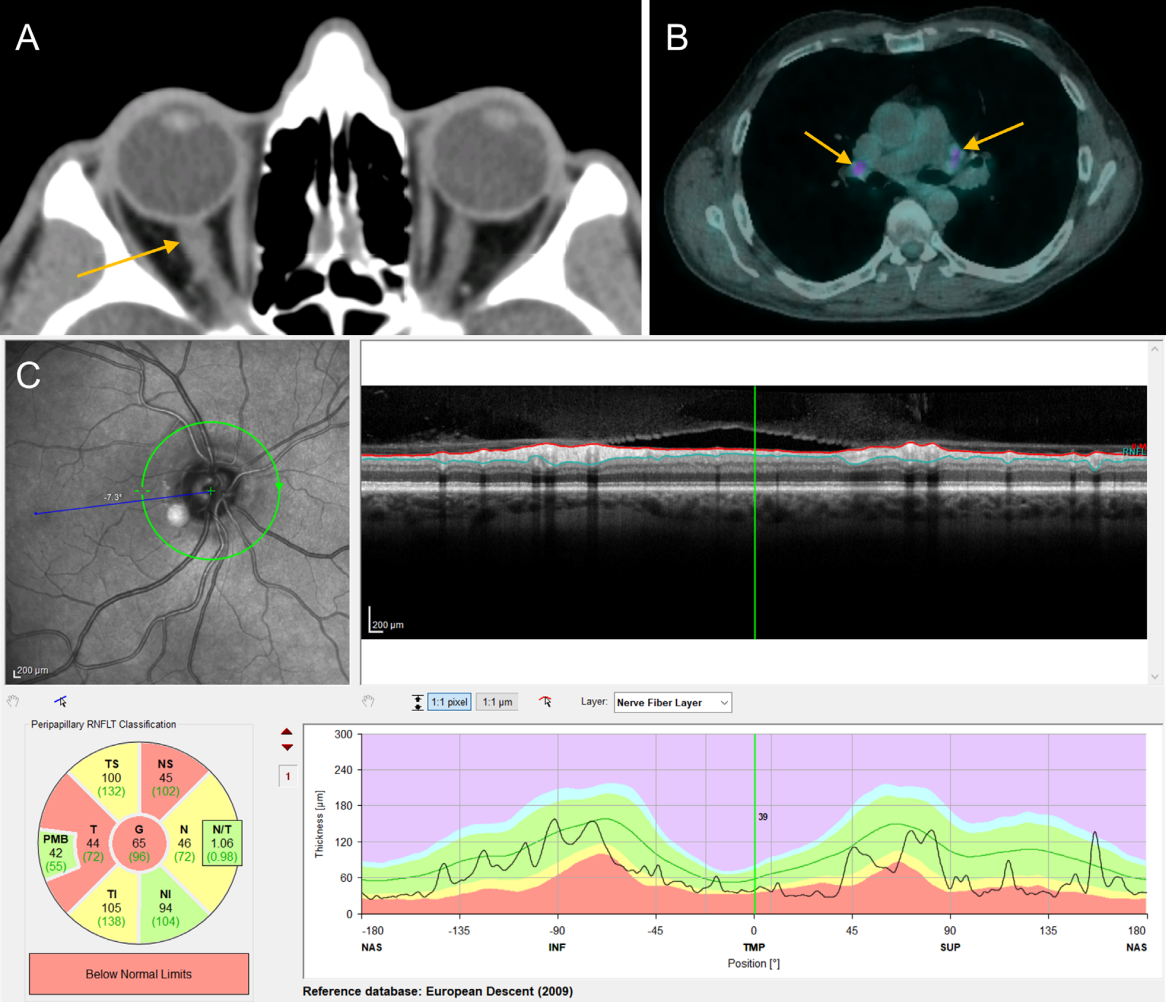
(A) CT head demonstrating asymmetrical enlargement of the optic nerve sheath complex within the right orbit (arrow); (B) FDG‐PET with FDG‐avid hilar lymphadenopathy (arrows); (C) OCT of the right eye, demonstrating supero‐temporal thinning of the peripapillary retinal nerve fibre layer.
